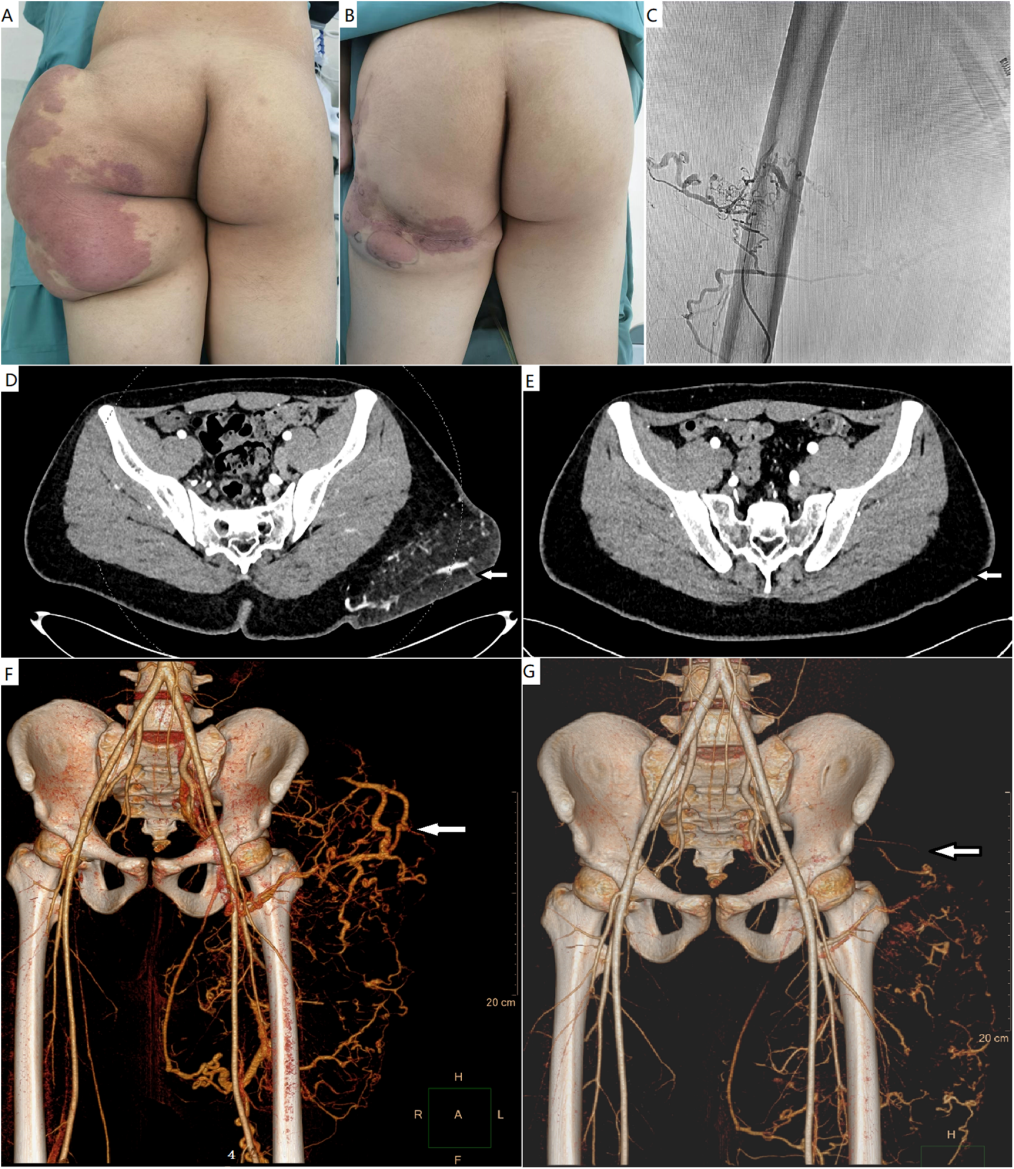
(A) A male patient with a CAVM with massive fatty overgrowth in the left buttock. (B) He underwent debulking surgery. (C) One year after surgery, ethanol embolization was performed to ablate the residual CAVM. (D,E) Pre- and post-procedural computed tomography studies with contrast enhancement. The mass with AVM was successfully debulked. (F,G) Pre- and post-procedural computed tomography angiography showing the markedly ablated vascular component. CAVM, capillary arteriovenous malformation; AVM, arteriovenous malformation.
